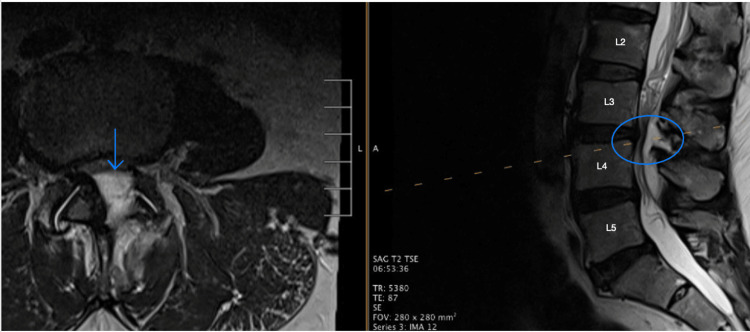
Preoperative MRI indicating the central canal and lateral recess stenosis at L3-4. Shown in blue is the original pathology: central canal and lateral recess stenosis at L3-4, which is responsible for the patient's symptoms. MRI, magnetic resonance imaging
